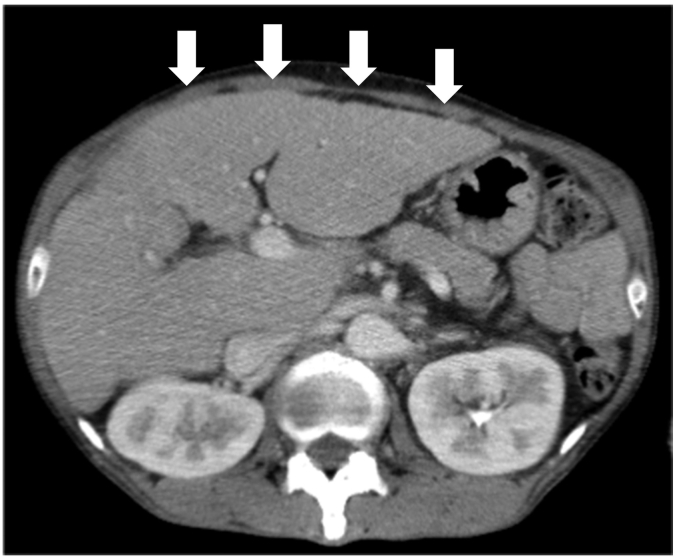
Enhanced computed tomography image. Irregularities are seen on the surface of the liver, suggestive of liver cirrhosis (arrows).
